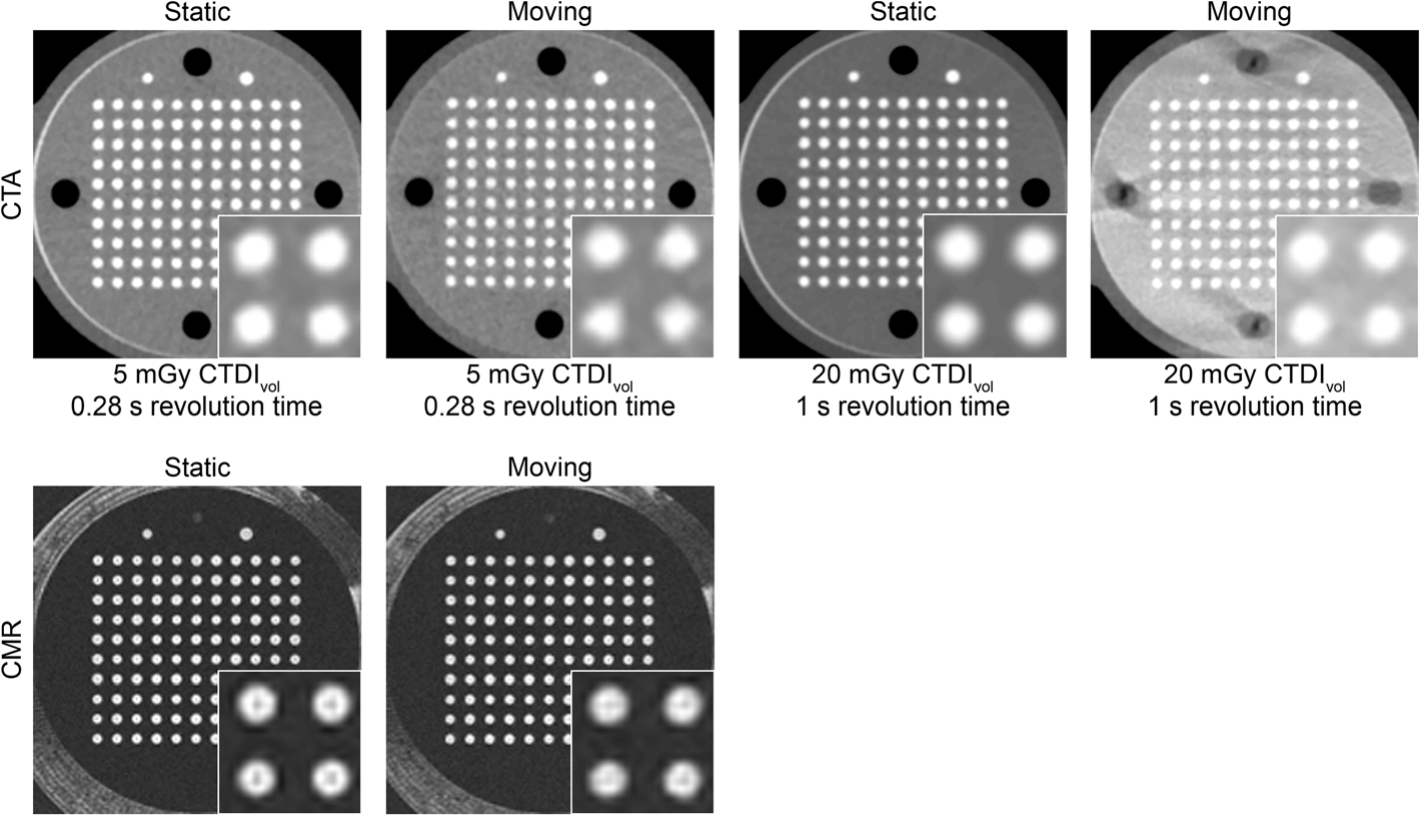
Comparison of representative computed tomography angiography (CTA) and cardiovascular magnetic resonance (CMR) images obtained under static and moving conditions. CTA data were acquired using 5 or 20 mGy (with adaptation of the gantry revolution time), 100 kVp, and 6% iodine, and reconstructed with 90% ASiR-V. CTDI_vol_ indicates volume CT dose index
